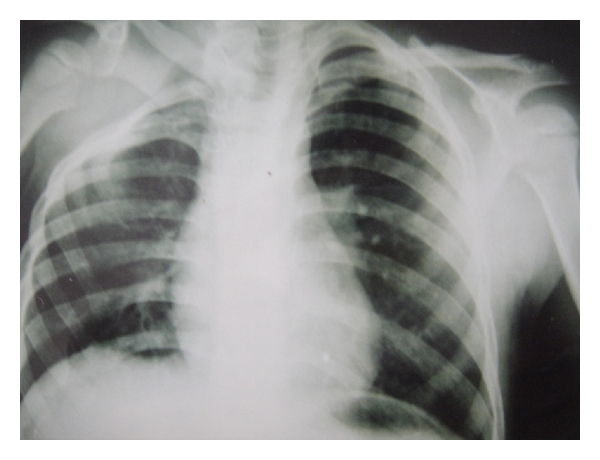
Anteroposterior radiography. This radiography shows right Sprengel's deformity associated with costal anomalies. Scapulovertebral bone is not seen.
